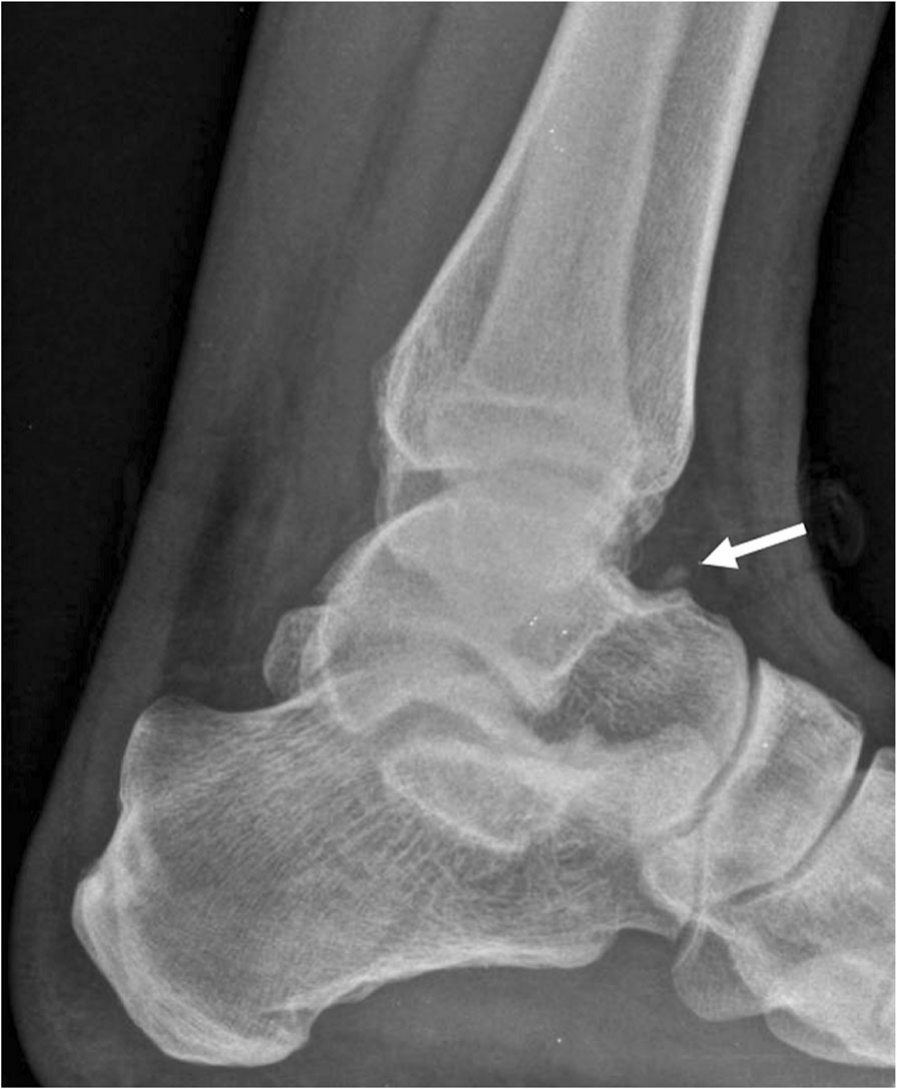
40-year-old man, incidental finding of a small os supratalare (white arrow), as well as an os trigonum. Patient was referred for radiographs with the suspicion of Achilles tendinopathy in the contralateral leg
